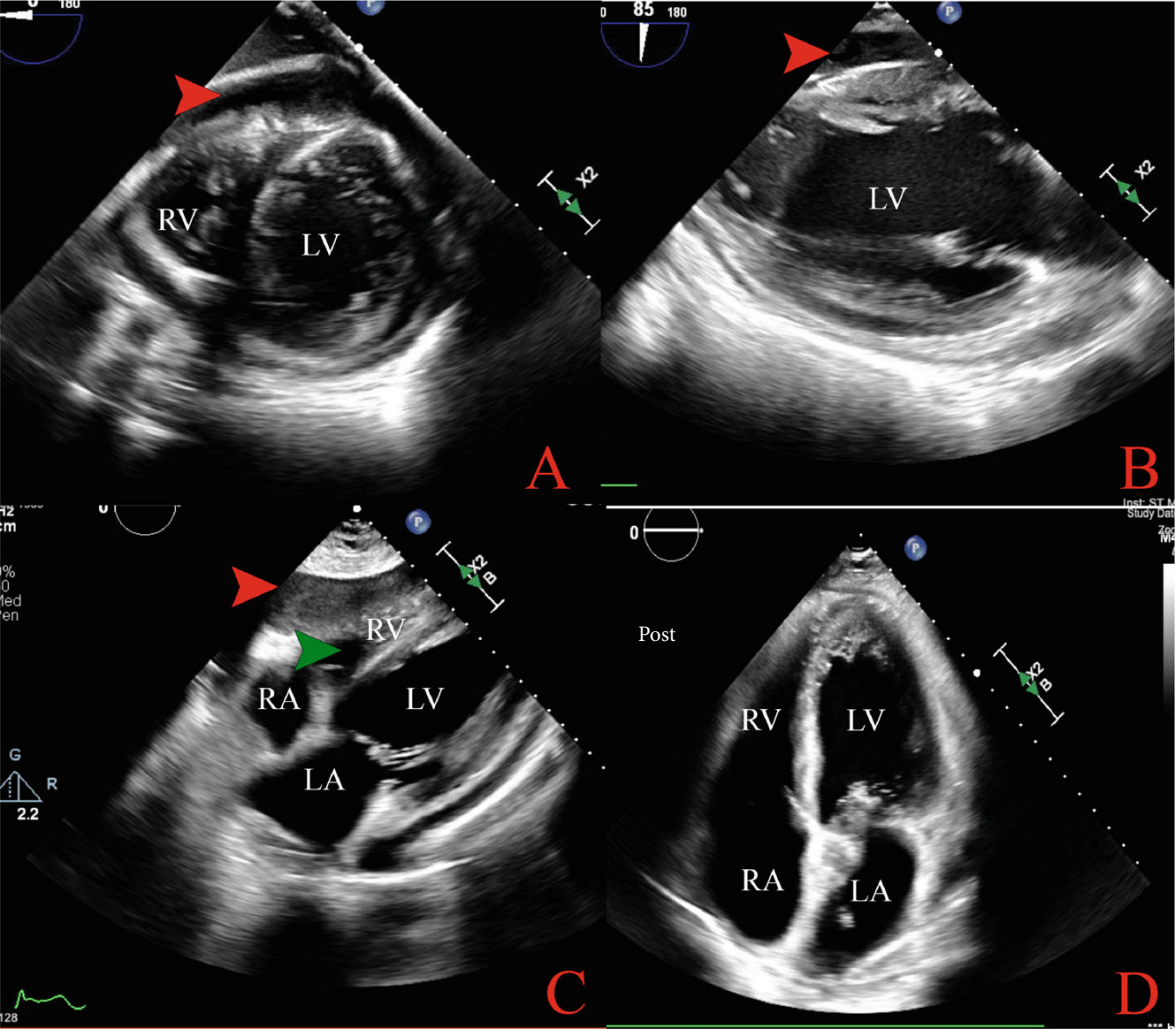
Echocardiogram. (A) Parasternal short-axis view showing large pericardial effusion (red arrow). (B) Parasternal short-axis view showing pericardial effusion (red arrows). (C) Parasternal short-axis view showing pericardial effusion (red arrow), evidence of RV collapse suggesting cardiac tamponade (green arrow). (D) Postpericardiocentesis apical four-chamber showing drained pericardial fluid. RA: right atrium, RV: right ventricle, LA: left atrium, LV: left ventricle.
